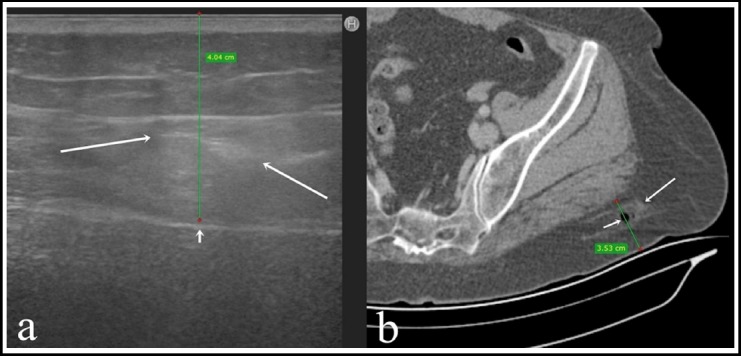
A 53-year-old female patient had diclofenac sodium injection. A) On US, dorsogluteal subcutaneous fat tissue (SAT) thickness was not appropriate for IM injection (green line:SAT thickness: 40.4 mm, short arrow: muscle fascia); drug is observed in SAT (long arrow). B) The patient also underwent CT scan immediately after the US examination. The CT scan revealed SAT injection densities (long arrow) and incorrectly injected air within these densities (short arrow). On CT, dorsogluteal SAT thickness was 3.53 mm, the body weight of patient lying in the supine position on the patient couch during CT may have altered the location and thickness of SAT (green line: SAT).
